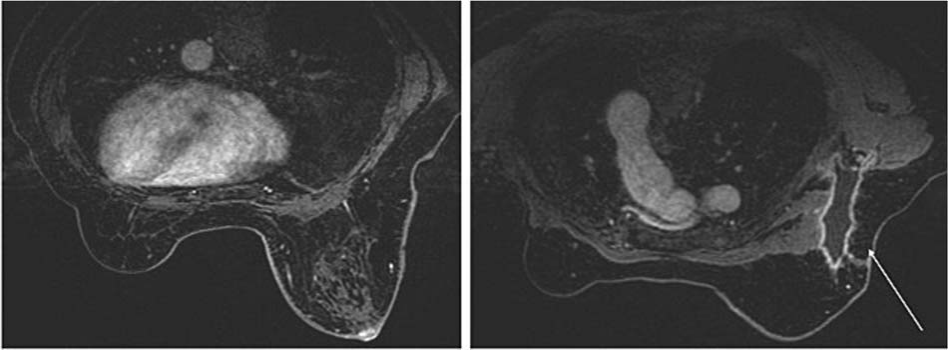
Breast magnetic resonance imaging (MRI) findings. No lesions were observed in the right breast, and a lymph node enlarged to 46 × 34 mm was observed in the right axilla.
